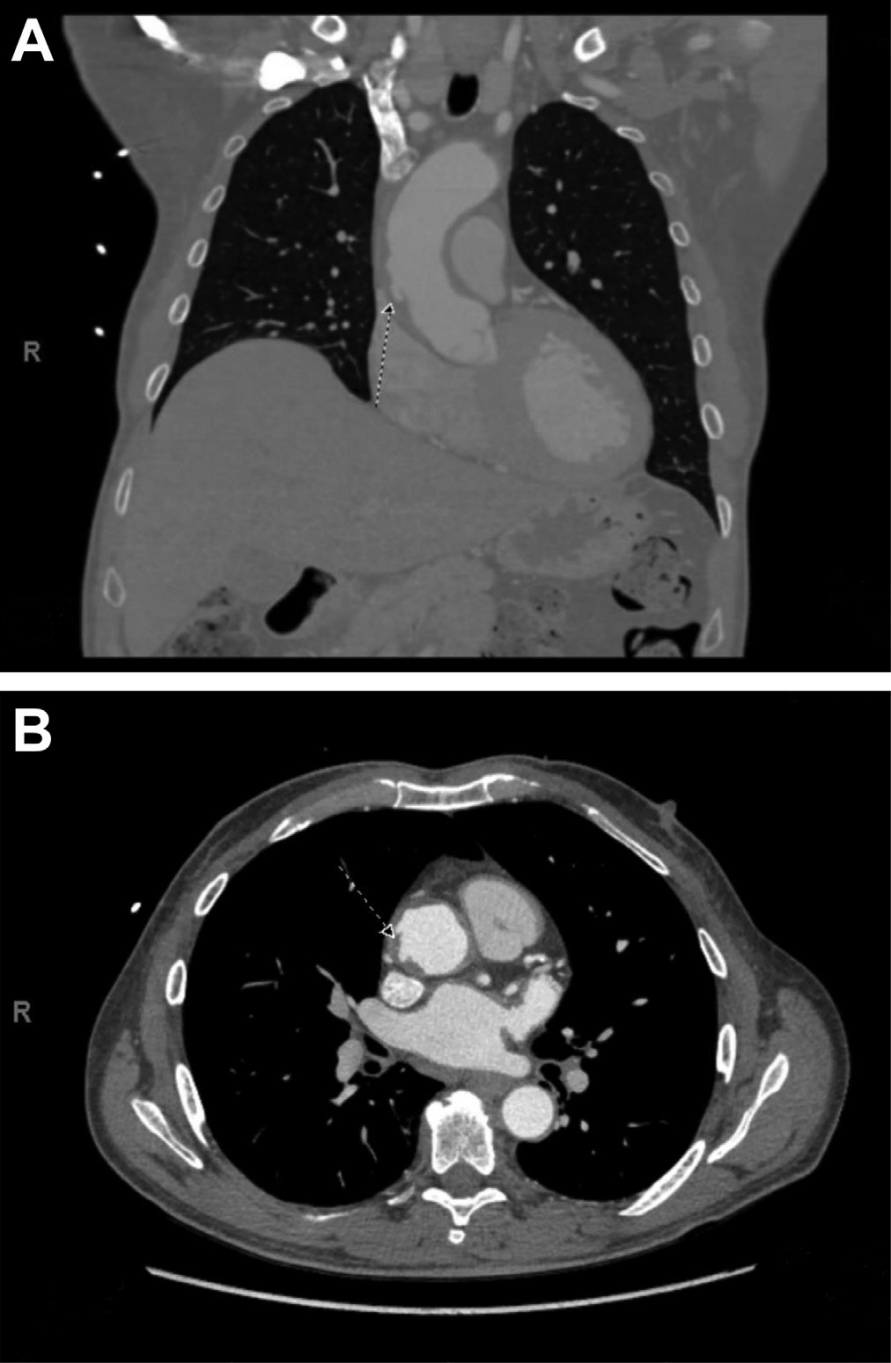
Computed tomographic angiograms in the A) coronal and B) axial views
